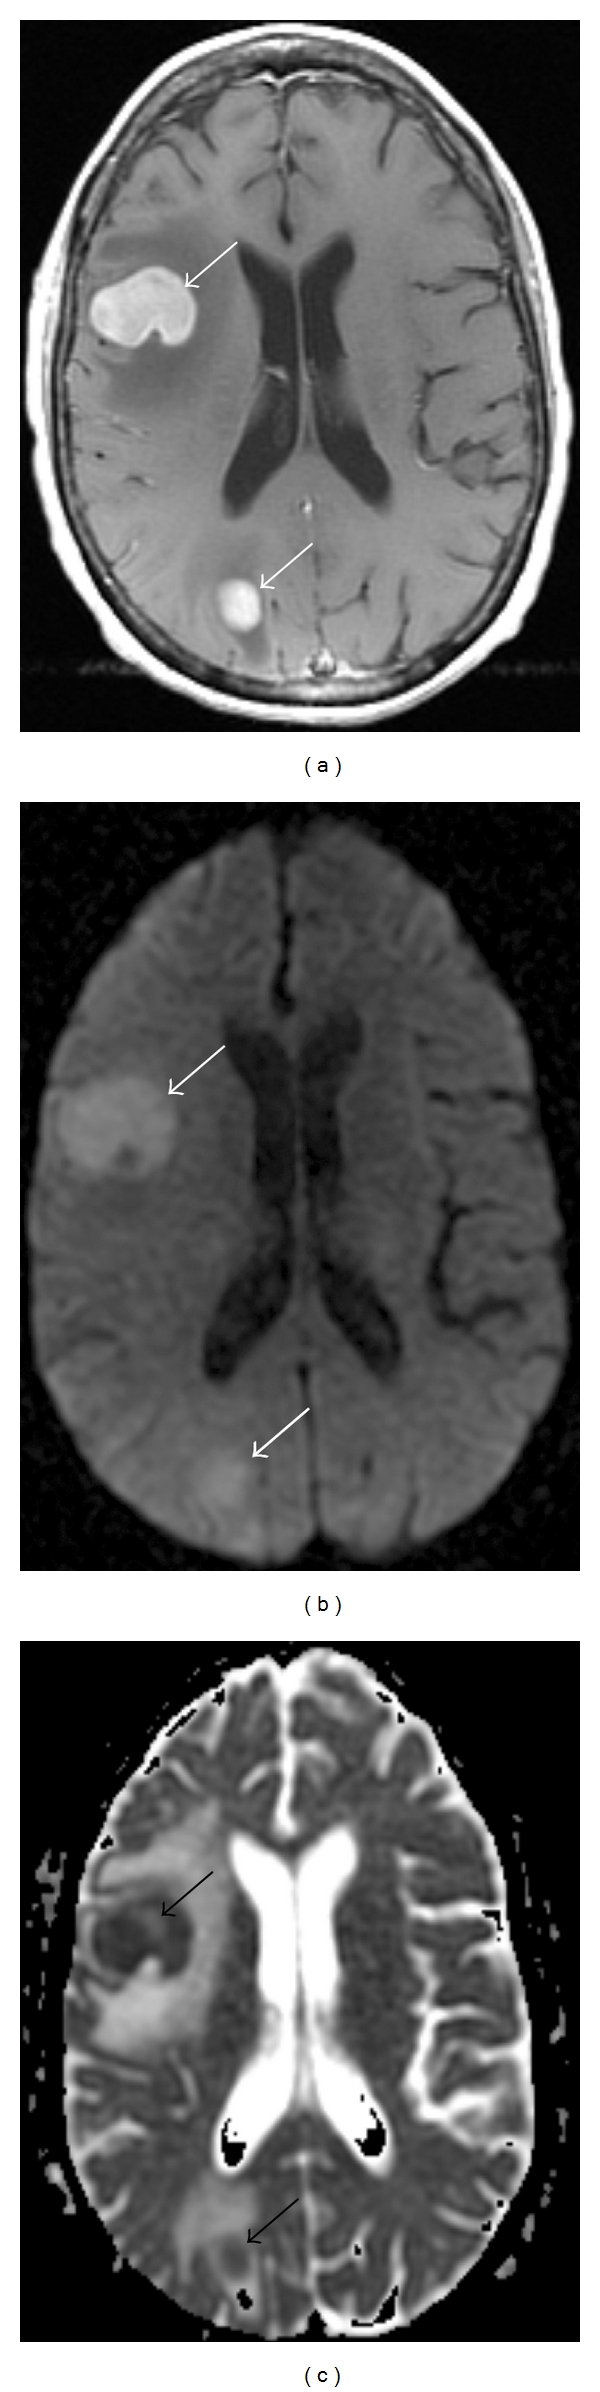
A 63-year-old female with diffuse large B-cell lymphoma involving the frontal and parietal lobes. (a) Axial T1 postcontrast. Diffusely enhancing right cerebral mass lesions are present (white arrows). (b) DWI sequence. The lesions are hyperintense on diffusion-weighted imaging (white arrows). (c) ADC map. The ADC map shows hypointensity of the lesions (dark arrows).
